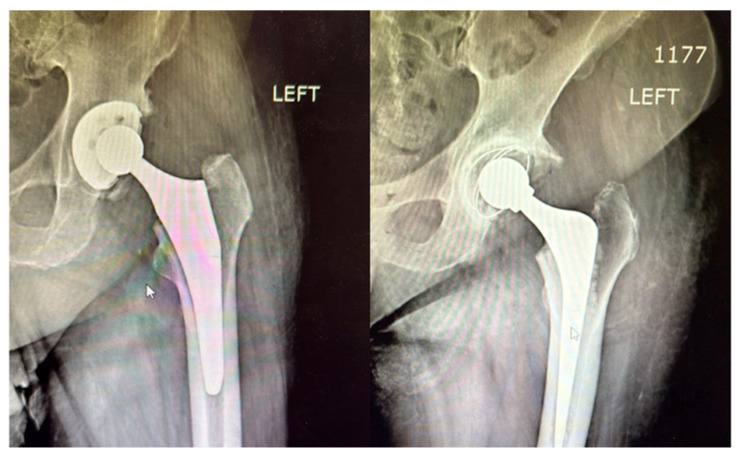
Postoperative radiographic comparison between cemented (right) and uncemented (left) femoral components in THA. The cemented stem (right) is outlined by a radiopaque cement mantle, while the uncemented stem (left) shows a press-fit alignment without visible cement, relying on bone integration for long-term stability.
